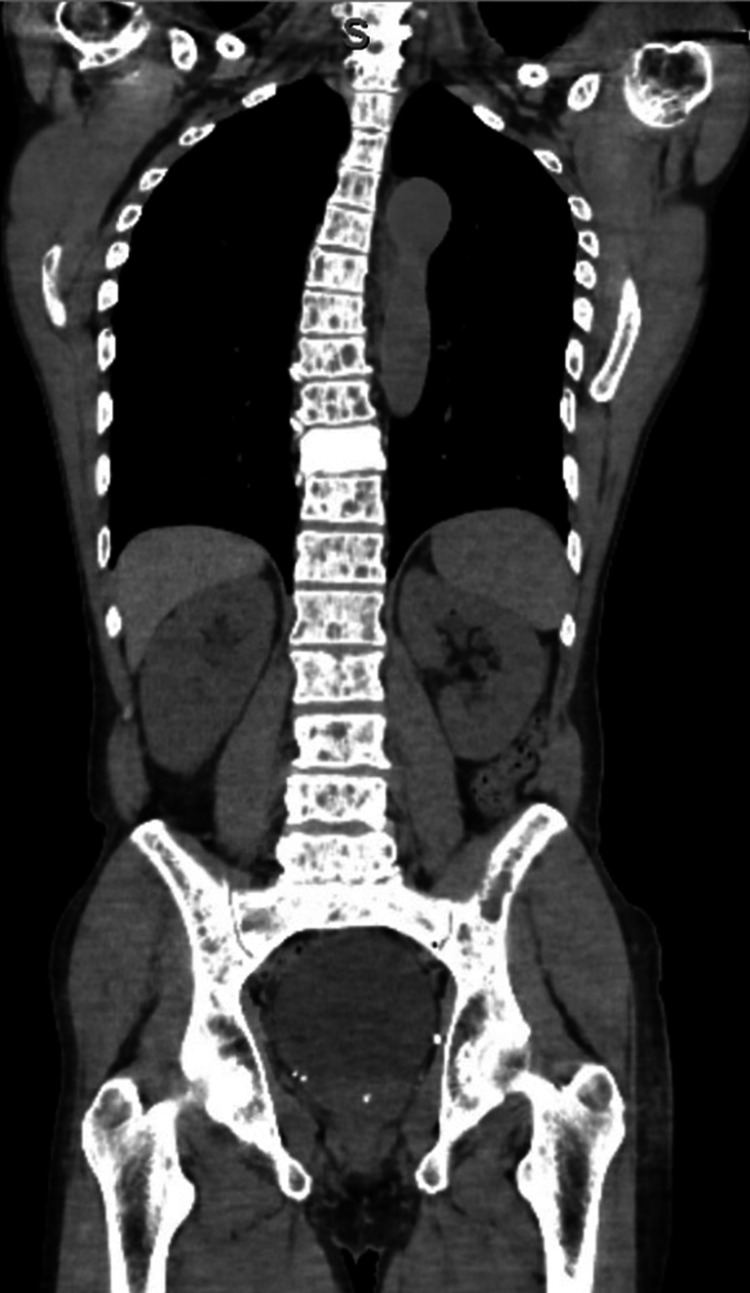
Coronal CT scan showing lytic lesions in the axial skeleton
